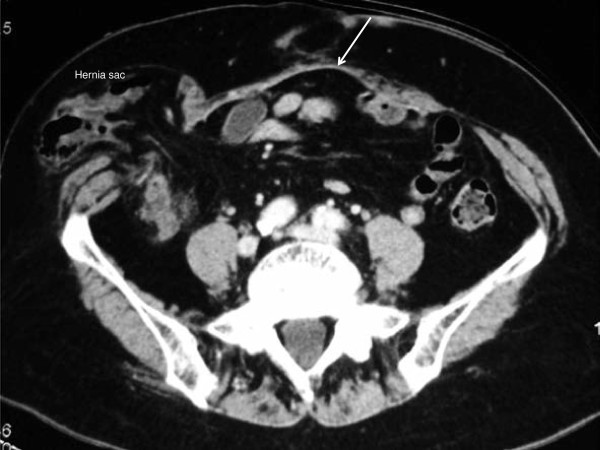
Preoperative computed tomography image of case 1. The transverse colon is protruding into the hernia sac lateral to the ileal conduit. A prosthetic mesh (arrow) previously used for a midline incisional hernia is visualized.
